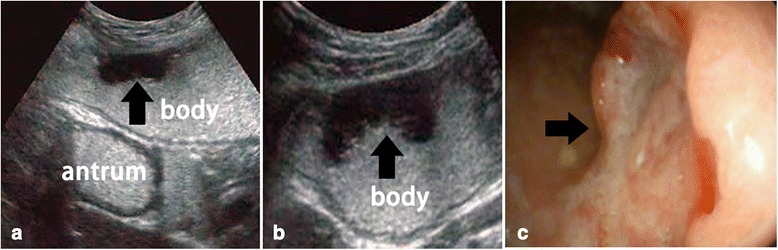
Advanced gastric cancer. a and b Examination involving transabdominal ultrasound scans after oral administration of an echoic cellulose-based gastric ultrasound contrast agent showing hypoechoic thickening of the gastric wall with superficial ulceration (a, longitudinal section; b, cross section). c Gastroscopic examination showing the lesion. Arrow, pointing at the lesion
